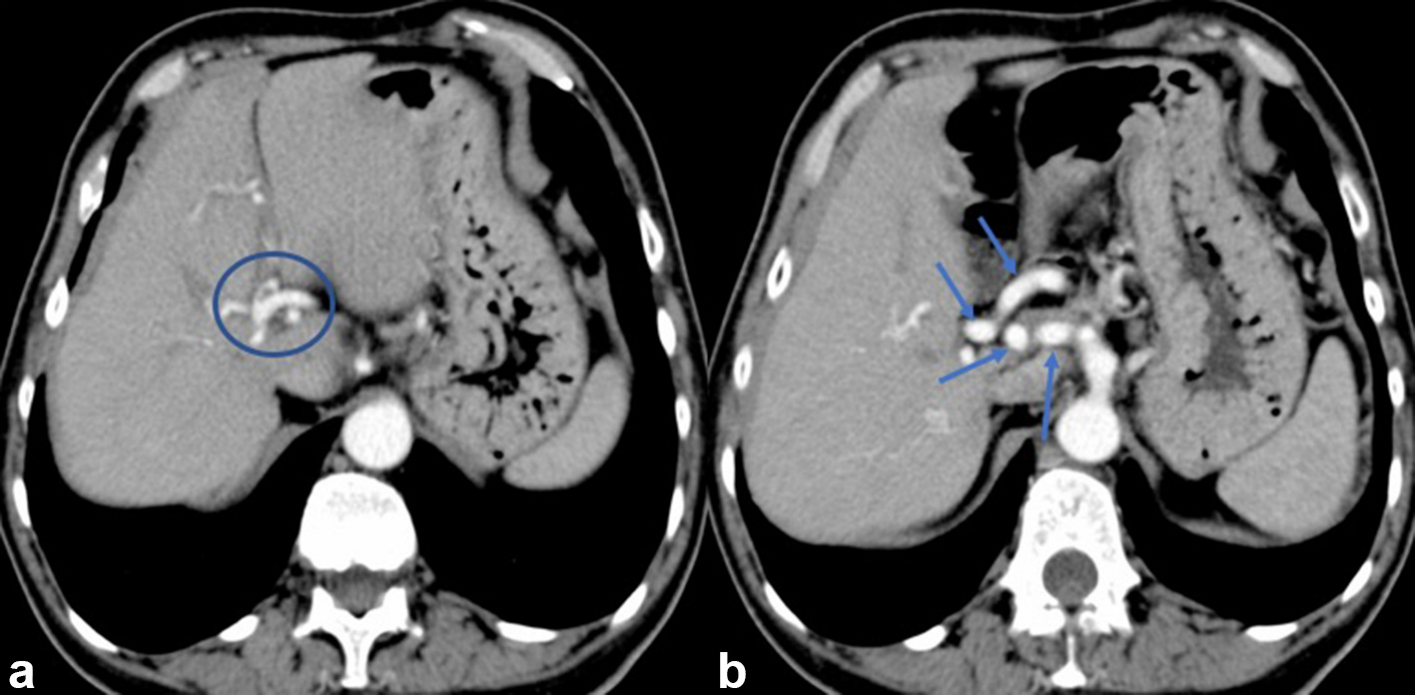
Axial CT images acquired during the arterial phase show a dilated (circle in a) and tortuous hepatic artery (arrows in b).
